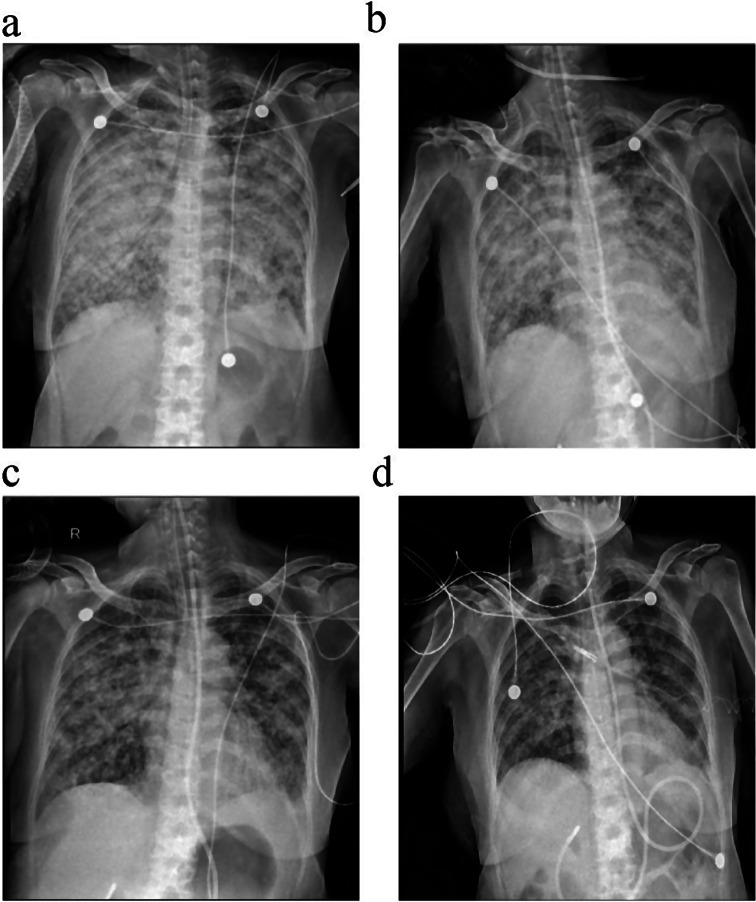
Dynamic changes in chest X-rays before and after treatment. (a) Multiple plaques in both lungs on January 13, 2024; (b) Minor reduced plaques on January 17, 2024; (c) Partial remission on January 21, 2024; (d) Significant remission on January 26, 2024
